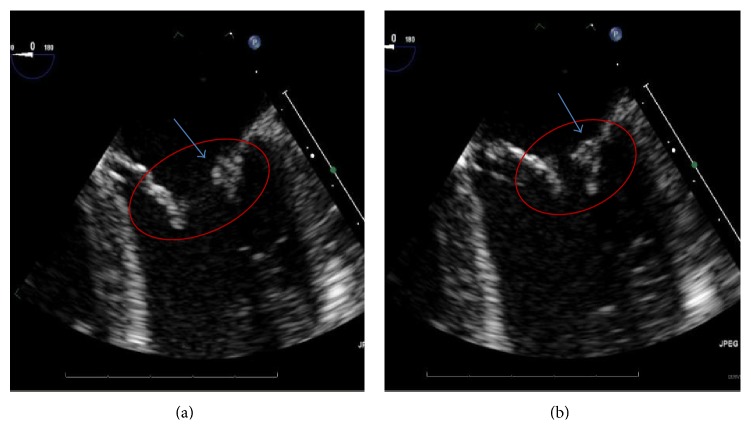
((a) and (b)) A cardiac transesophageal echocardiogram revealing severe mitral regurgitation and thickening of mitral valve leaflets with mitral valve leaflets not coapting and a small mass on the atrial side of the anterior mitral valve leaflet (arrow) suggesting Libman-Sacks endocarditis.
